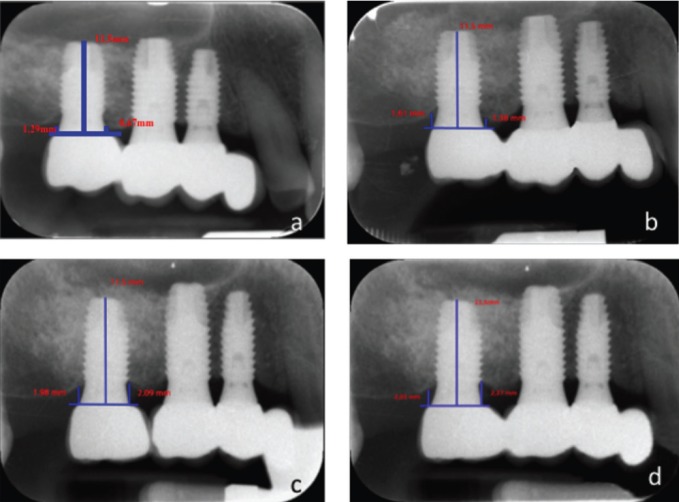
Periapical X rays showing the measurement of bone crest level both mesial and distal at the different study timepoints. Bone loss is recorded as the difference between the different moments over follow-up and prosthetic loading. a) Prosthetic loading; b) 12 months after loading; c) 5 years after loading; d) Maximum follow-up, 8 years after loading.
